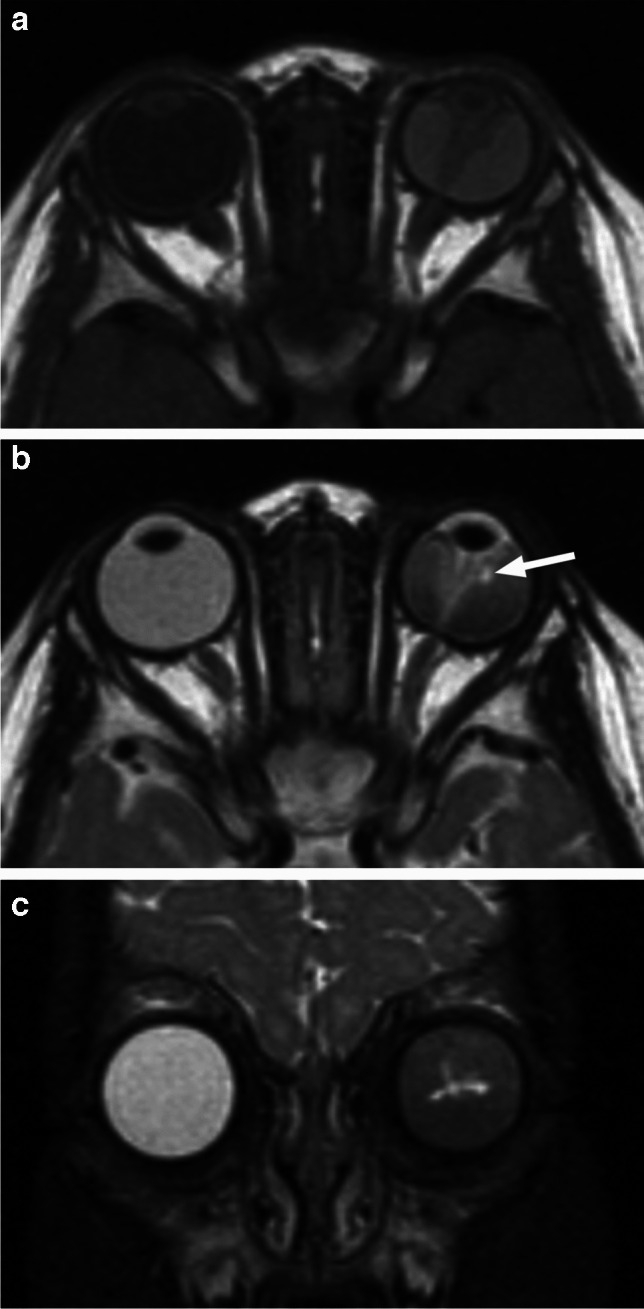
Coats disease. Axial T1-weighted image of the orbits (a) shows retinal detachment in the smaller left globe with hyperintensity of the exudate. Corresponding T2-weighted image (b) demonstrates low signal of the subretinal fluid and a retinal cyst (arrow). Extensive retinal detachment without evidence of an underlying mass in the smaller left globe is also seen on coronal STIR image (c)
